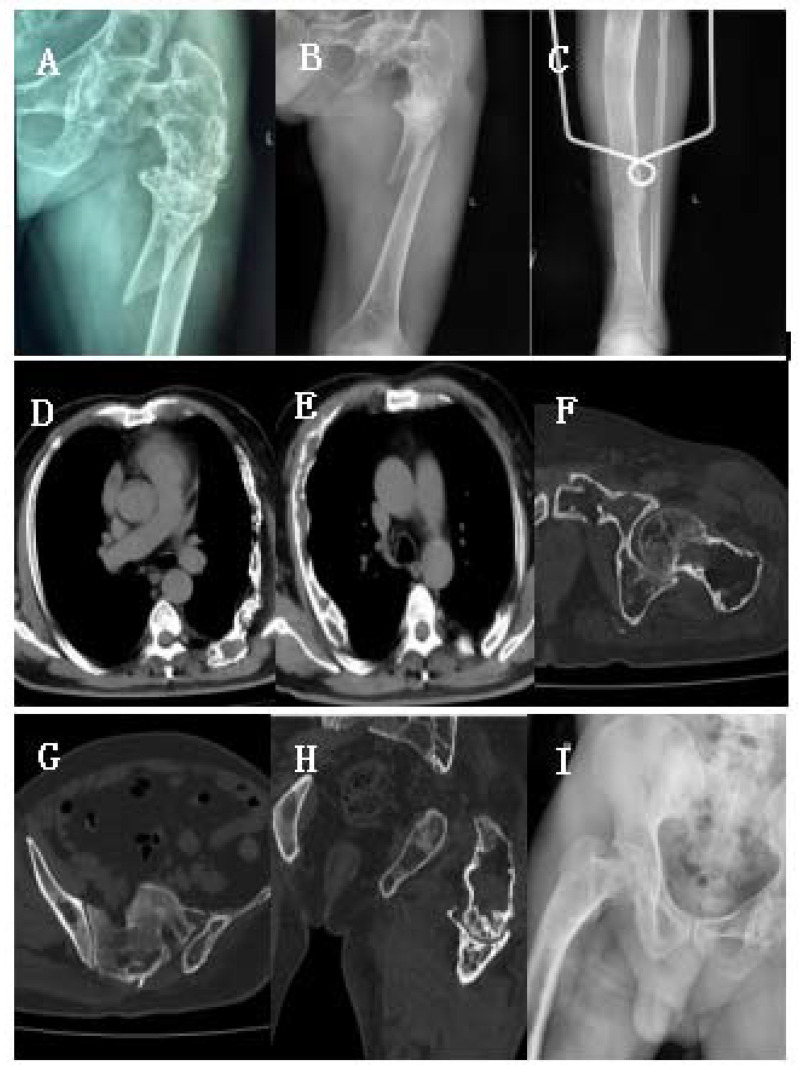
(A–I) Preoperative clinical imaging data. X-ray and CT showed fractures at the proximal end of the left femur, shepherd's crook deformity, and multiple fibrous dysplasia in the femur, ribs, acetabulum, and tibia.
